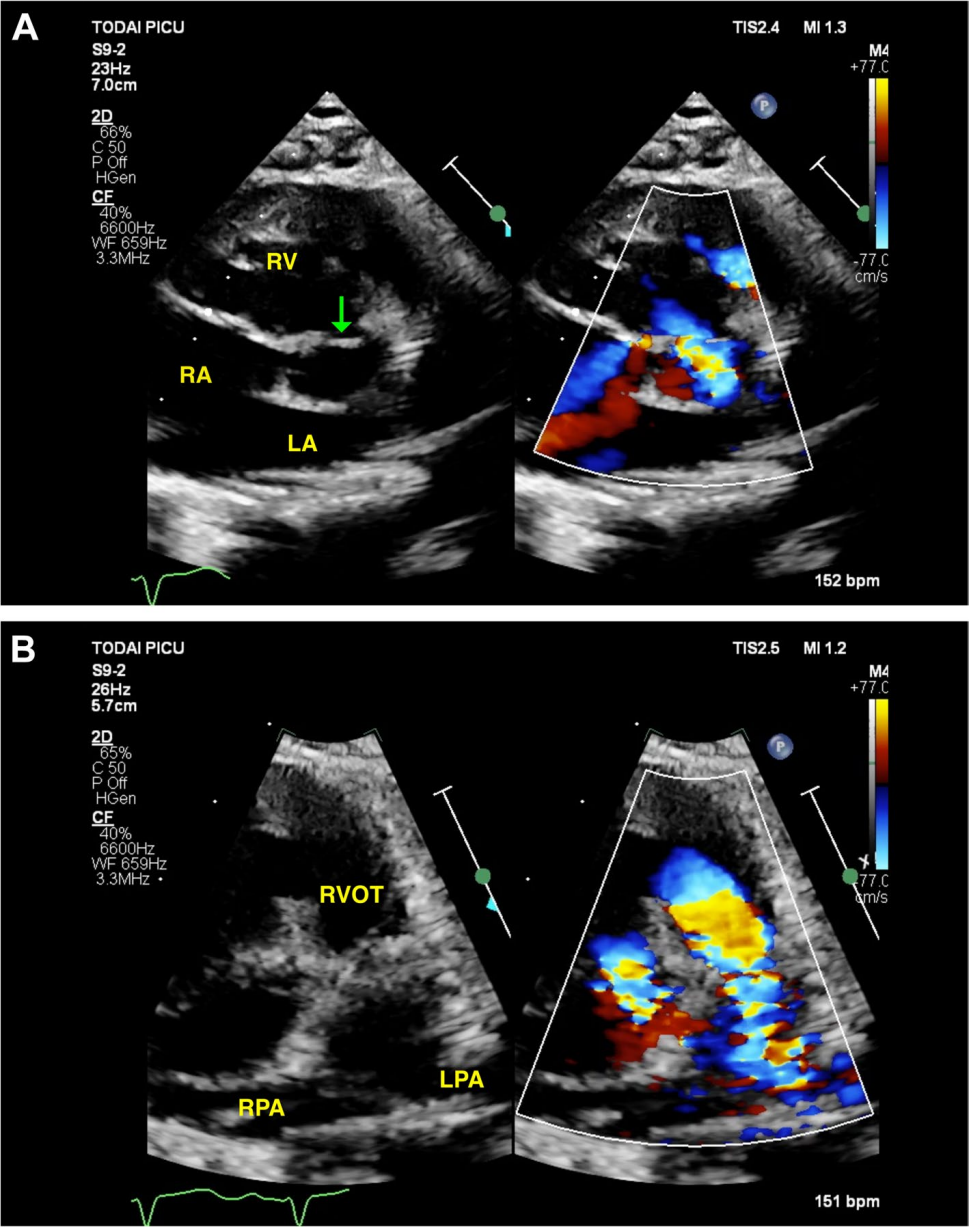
Preoperative Doppler echocardiography. A Parasternal short-axis view showed restrictive ventricular septal defect (VSD) caused by flap-like tissue in continuity with the tricuspid valve (green arrow), that is, accessory tricuspid valve, with right-to-left shunt and with a gradient of 61 mmHg, suggesting suprasystemic right ventricular pressure. B Parasternal short-axis view showed severe pulmonary stenosis with a peak right ventricular outflow tract (RVOT) gradient of 149 mmHg, which also suggested extremely high right ventricular pressure
